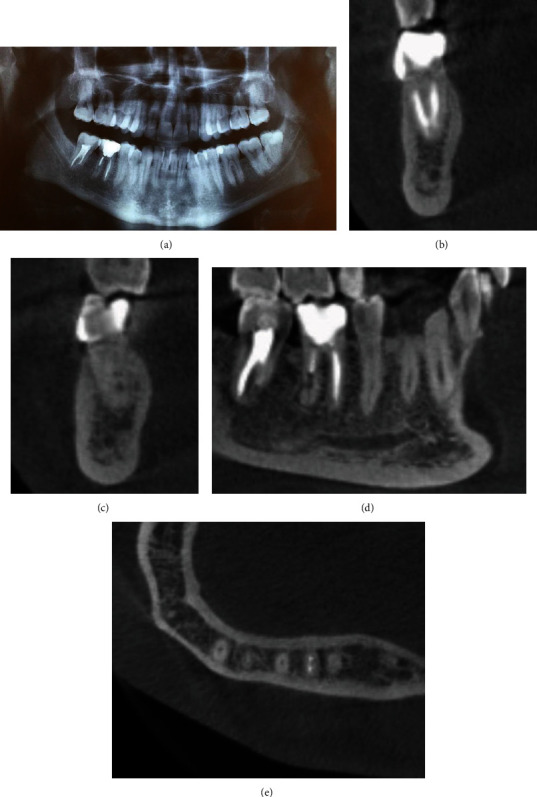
Panoramic radiograph showing complete healing and absence of recurrence after five years. (b–d) Five-year CBCT examination showing complete healing of the lesion. (c–e) The sagittal and axial views demonstrate remineralization of the area around and within the perforated root and depict the complete formation of the vestibular and lingual cortical plates.
